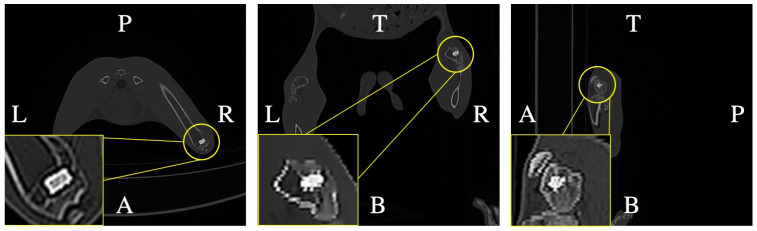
Postoperative computed tomography. The scaffold position was defined by postoperative computed tomography examination. The yellow frame shows a larger picture of the scaffold inside the femur.
